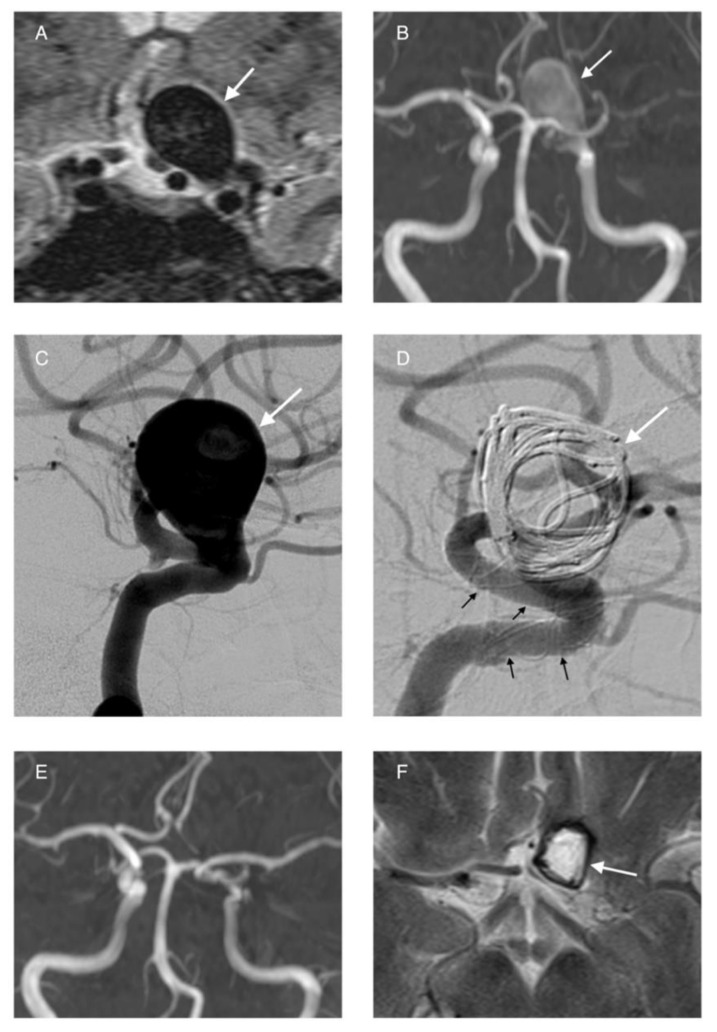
Giant aneurysm of the left ICA found on MRI and MR-angiography (A,B, white arrow) and clearly depicted with DSA (C, white arrow). The aneurysm was treated with implantation of a flow diverter (D, black arrows) and deposition of coils inside the aneurysm in order to induce thrombosis (D, with arrow). Follow up MRI and MRA show the completely occluded and thrombotic aneurysm (E,F, white arrow).
